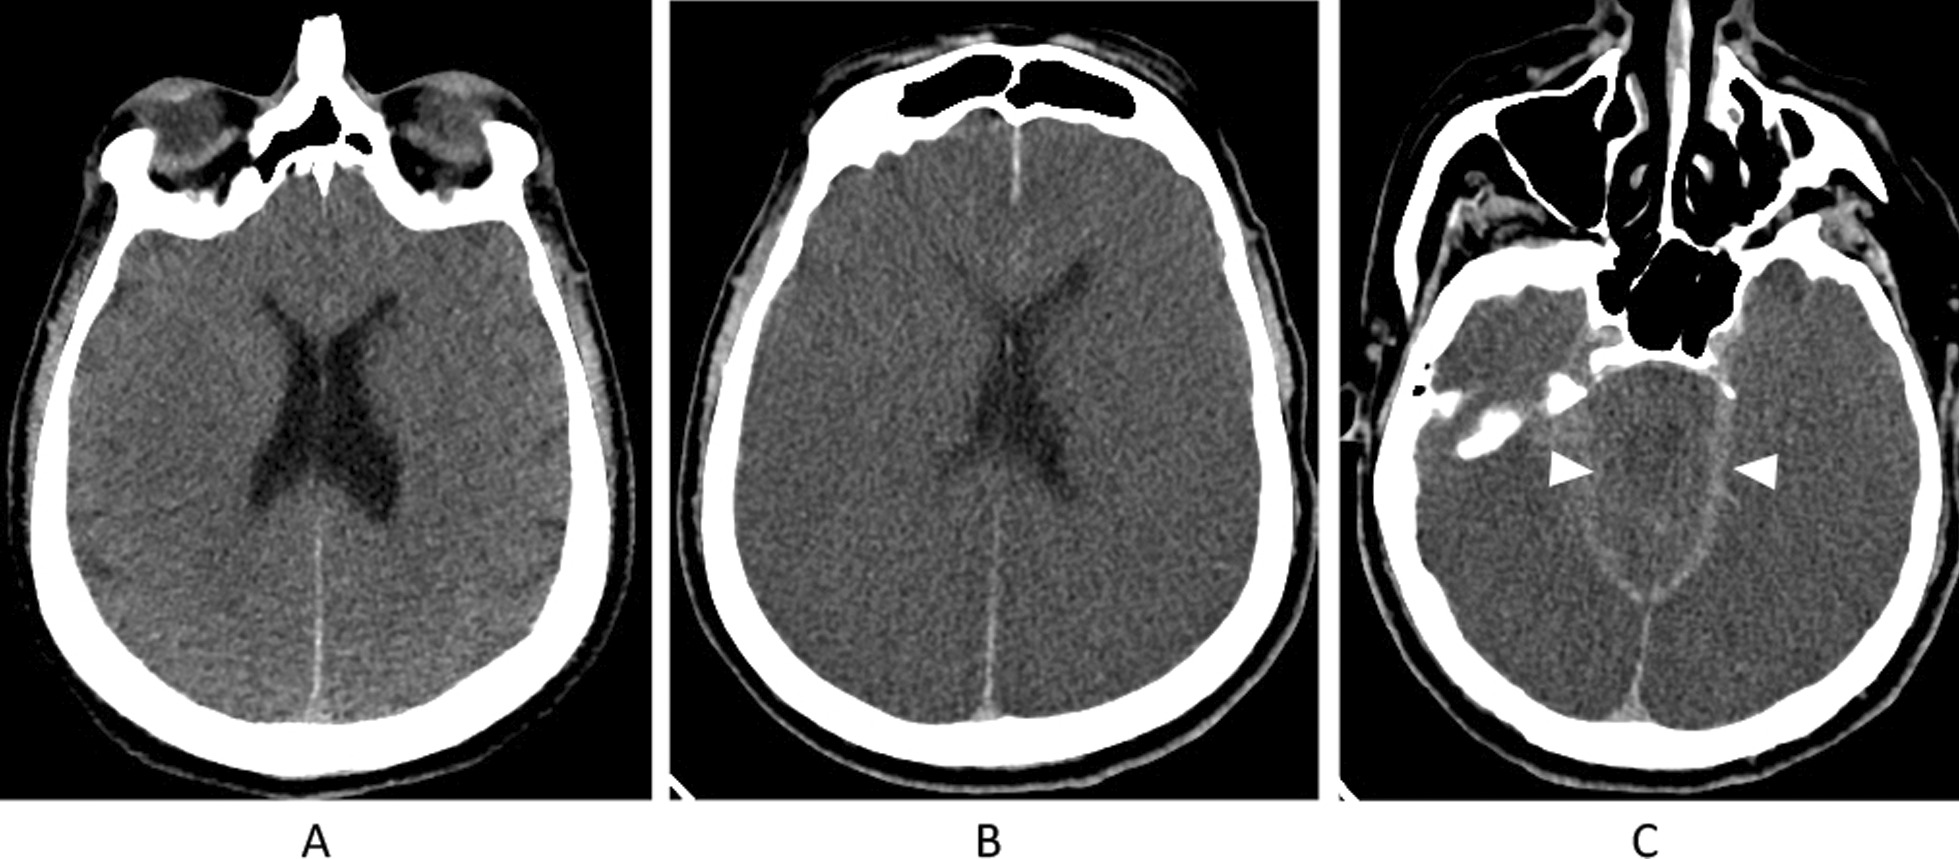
Imaging characteristics of catastrophic brain injury. Selected computed tomography (CT) images of a patient who presented to our hospital following cardiac arrest with anoxic brain injury. Initial non-contrast CT image obtained less than 2 h following initial arrest (A) demonstrates early loss of grey–white matter differentiation of the cerebral cortex. Follow-up study 36 h later (B and C) demonstrates progression of loss of grey white matter differentiation including the visualized brainstem with increased cerebral edema, sulcal and ventricular effacement and effacement of the basilar cisterns (arrowheads)
